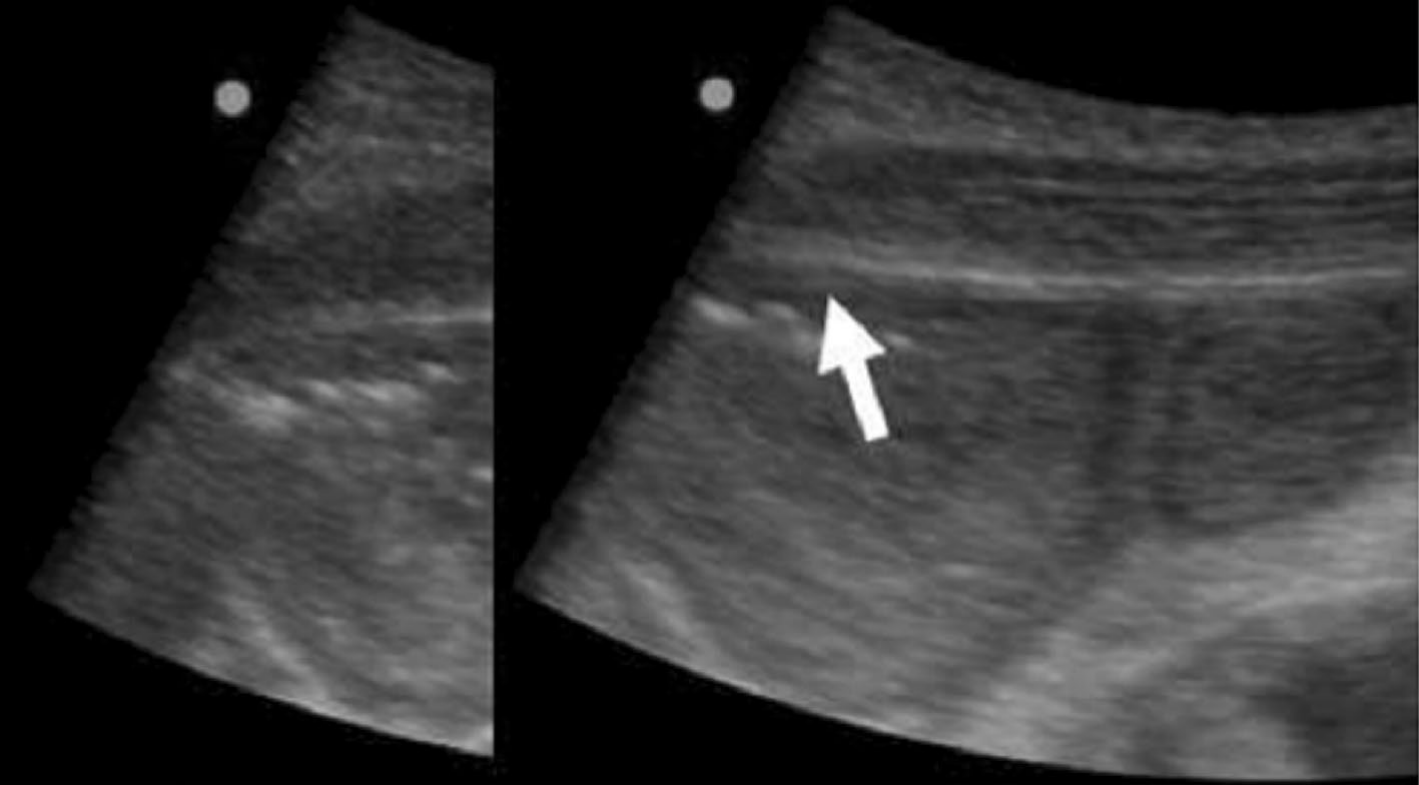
Peritoneal rebound visualized by ultrasound assessment in combination with external pressure. Loss of peritoneal rebound visualization happens when IAP is equal to or more than external pressure (adapted with permission from See et al. [26])
